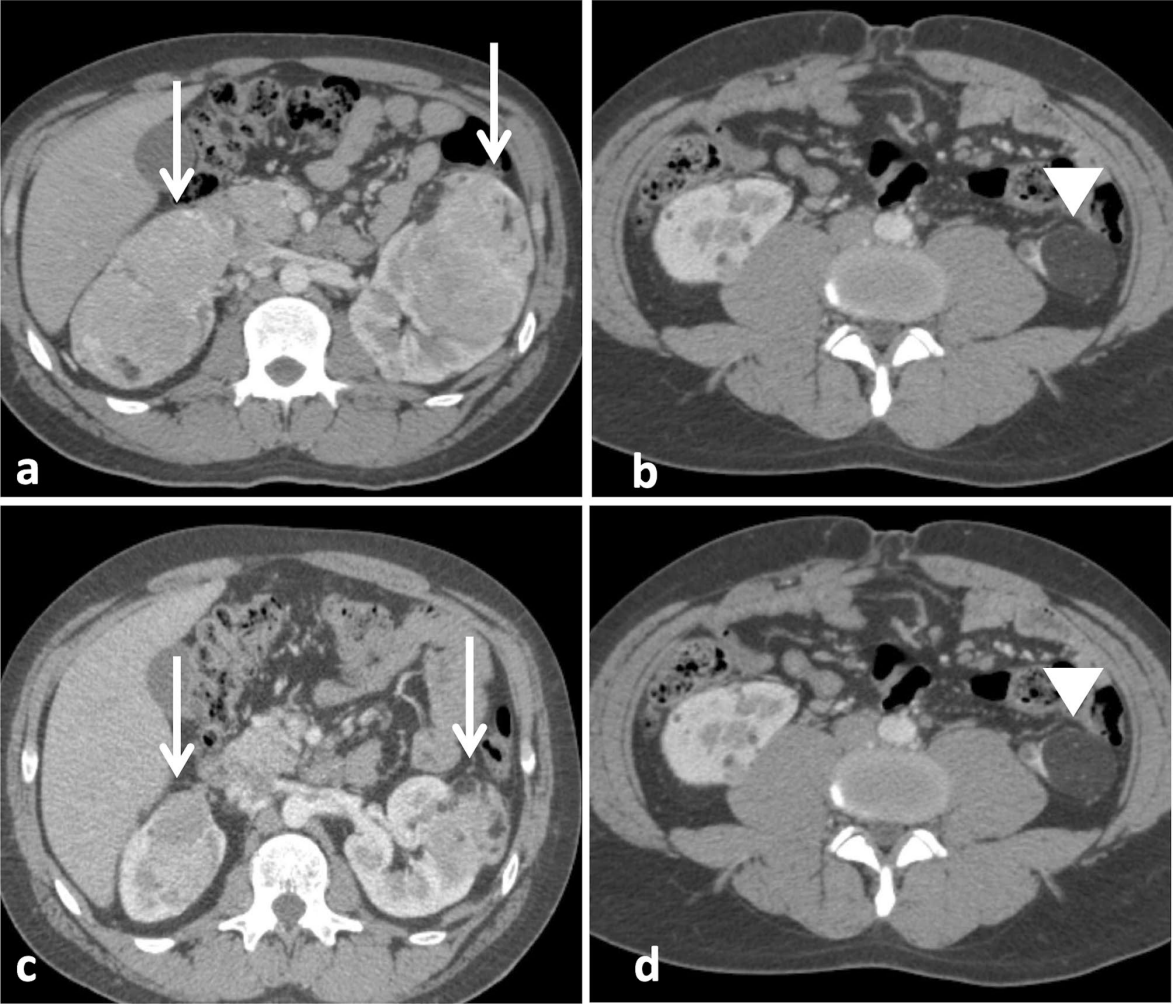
Response of renal AMLs to treatment with sirolimus. (a,b) Axial corticomedullary-phase CT images obtained before treatment show lobulated fat-poor AMLs in both kidneys (arrows) and a fat-rich AML arising from the lower pole of the left kidney (arrowhead). (c,d) Axial corticomedullary-phase CT images acquired after 7 months of treatment show significant volumetric reduction of the fat-poor AMLs (arrows) and nonsignificant change in the fat-rich AML (arrowhead).
